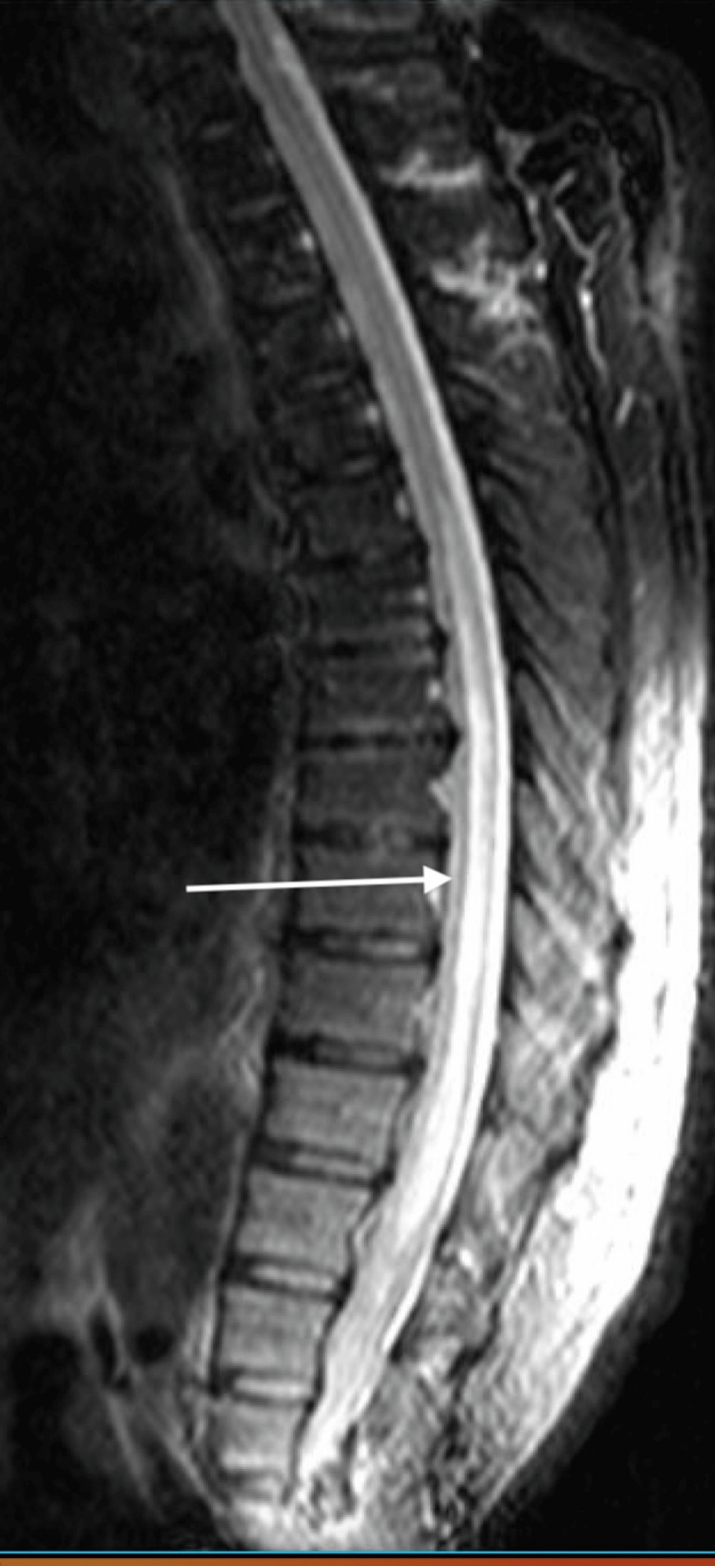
Magnetic resonance imaging of the thoracic spine with fat suppression showing hyperintensity from T9 to conus
